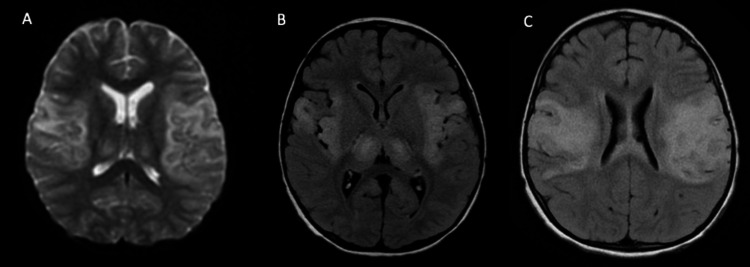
Brain MRI during the initial presentation. A: Axial DWI. B: Axial T2 FLAIR + GAD. C: Axial T2 FLAIR + GAD. DWI, diffusion-weighted imaging, FLAIR, fluid-attenuated inversion recovery, GAD, gadolinium.
